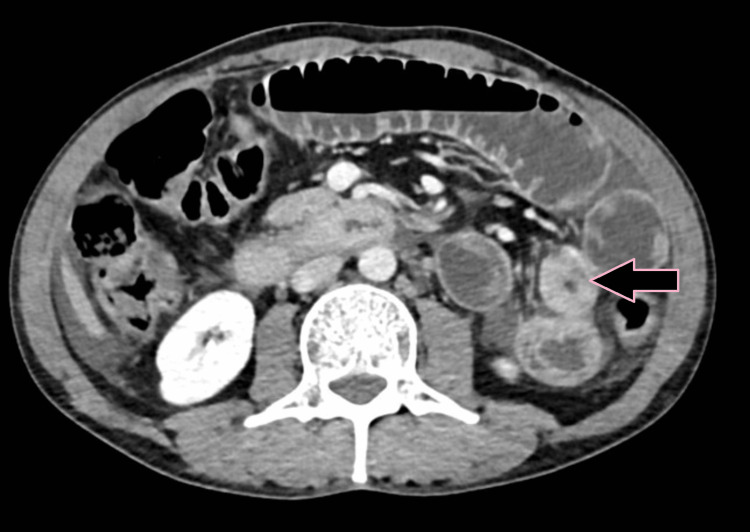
Axial CECT image showing circumferential bowel wall thickening of the jejunum (pink arrow) with a normal adjacent small bowel loop indicated for comparison (case 1). CECT: contrast-enhanced computed tomography
